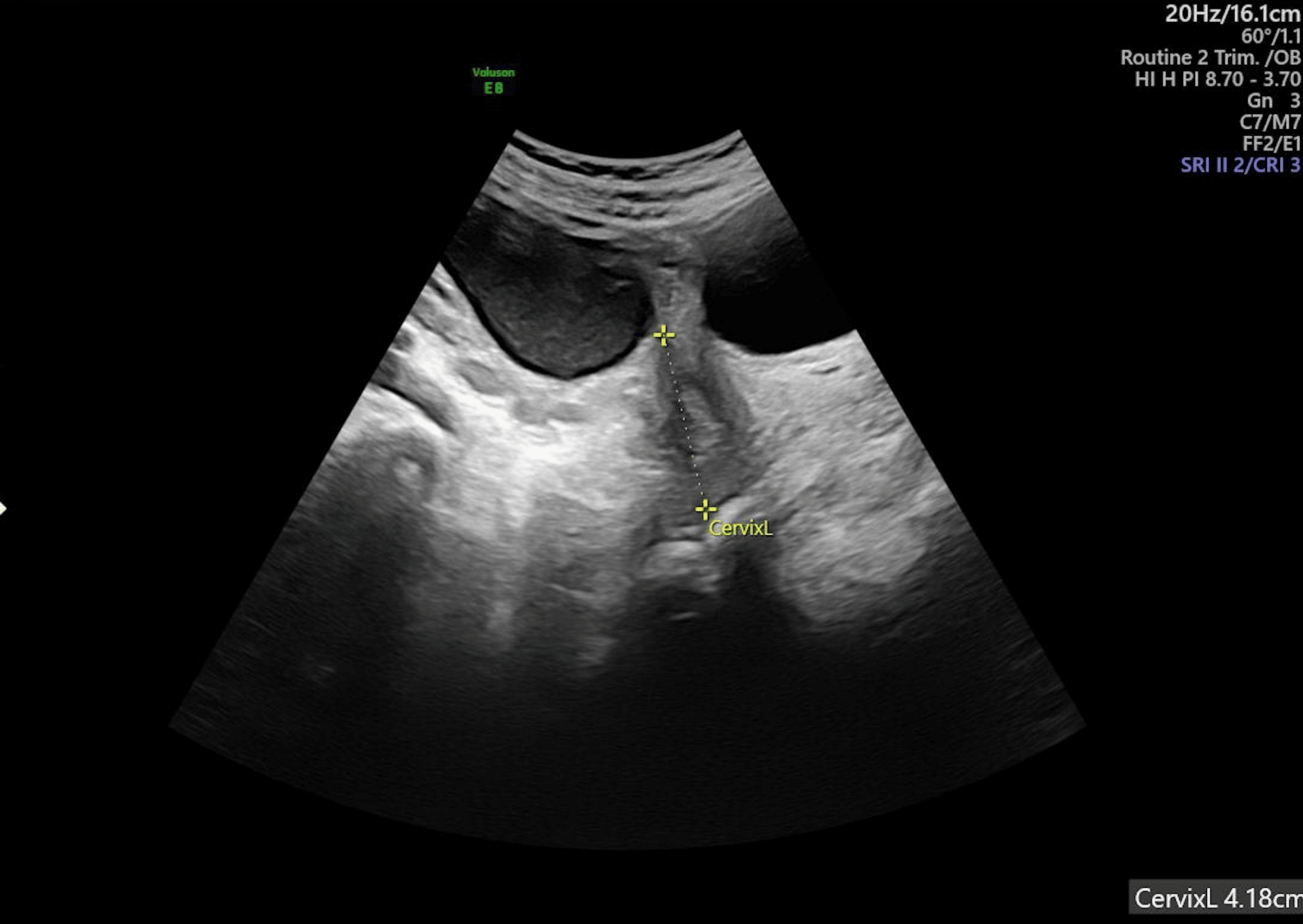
The patient’s midtrimester ultrasound at 23.1 weeks of pregnancy
